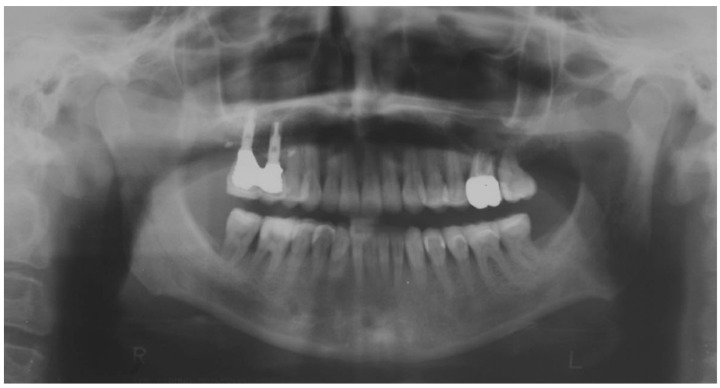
Orthopantomography at 24 months.
